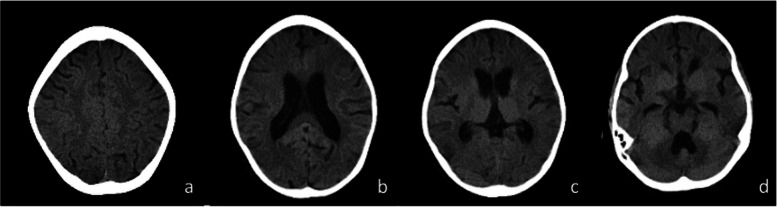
Head CT showed slightly lower parenchyma density in both cerebral hemispheres and an unclear intracranial gray matter boundary. Sulci slightly widened and deepened, bilateral lateral ventricles widened, posterior fossa pool widened
